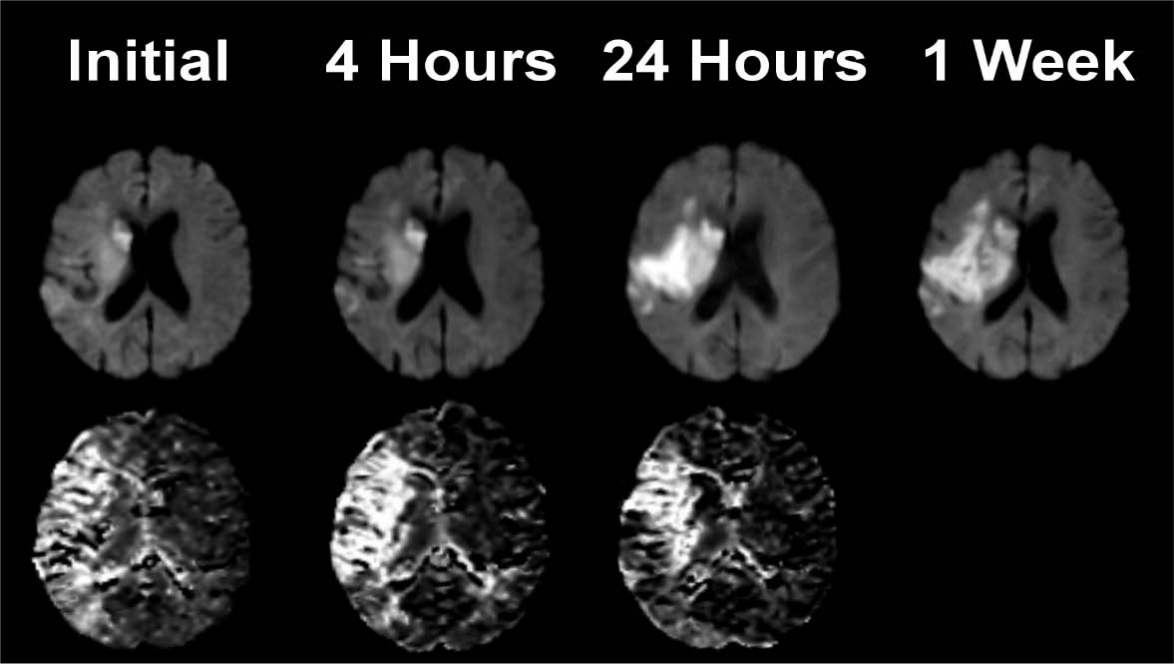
Imaging data from a patient with a left hemiparesis. Diffusion weighted images (top row) and mean transit time (bottom row) at the level of the lateral ventricles from a patient who presented 4.2 hours after onset of a left hemiparesis, and who was found to have a proximal right middle cerebral artery occlusion by CT angiography. Thrombolytic therapy was not given, but normobaric oxygen was provided by face mask for 4 hours. The image abnormalities were located within the right middle cerebral artery territory. The sizes of the diffusion and MTT abnormalities are very similar at the first 2 time points and there is a large mismatch. At 24 hours after baseline imaging, a large increase in the abnormal diffusion volume is apparent accompanied by a decrease in the abnormal MTT volume, but a greater than 20% mismatch persists. At 1 week, continued increase of the abnormal DWI is observed.
